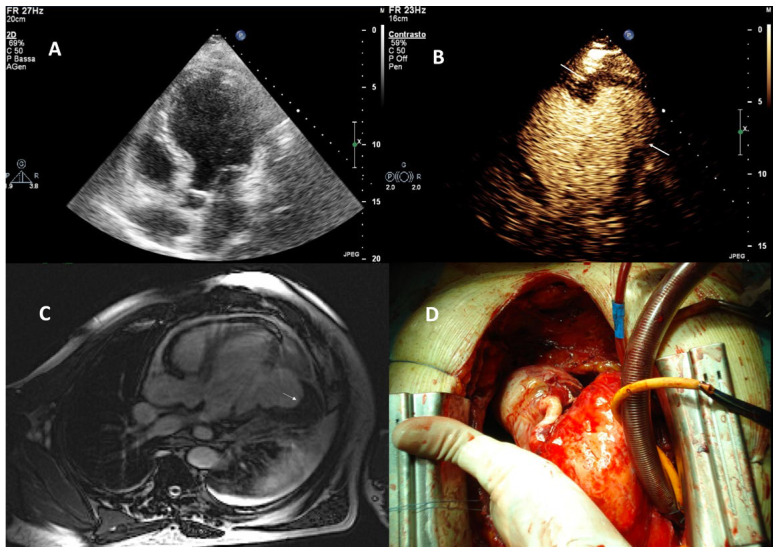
Large true aneurysm. Standard transthoracic echocardiography does not clearly visualize the post-MI lateral aneurysm (apical 4-chamber view) (A). After an intravenous bolus of UEA, CEUS reveals a massive lateral aneurysm (arrows) with suspect mural thrombus (apical 4-chamber view) (B). Cardiac magnetic resonance confirms the presence of the aneurysm and thrombus (arrow) (C). Intraoperative view of the aneurysm and thrombus (D).
